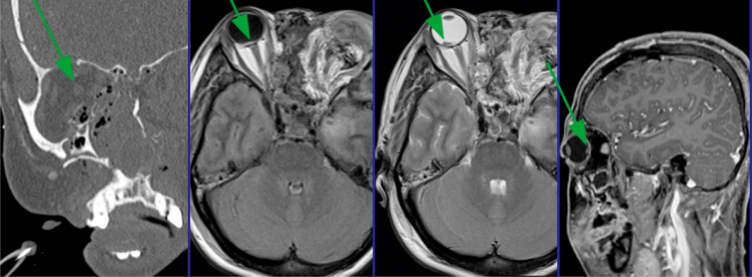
Avulsion injury. MRI: The arrows indicate bleeding into the location of rupture of the optic nerve (contralateral most severe contusion injury of the bulb).
